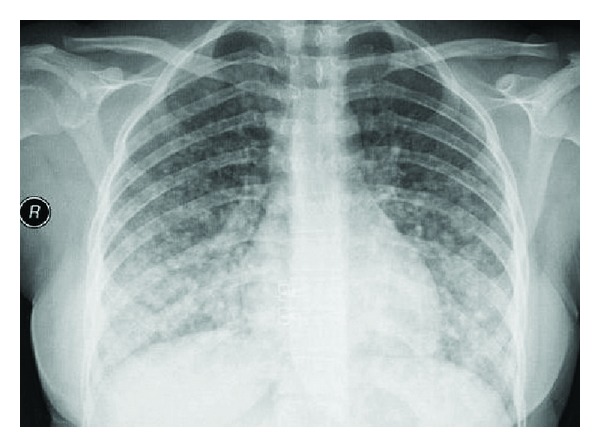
In the chest radiography, widespread nodular opacities are seen in all the zones, which are intensified in the lower zones.
